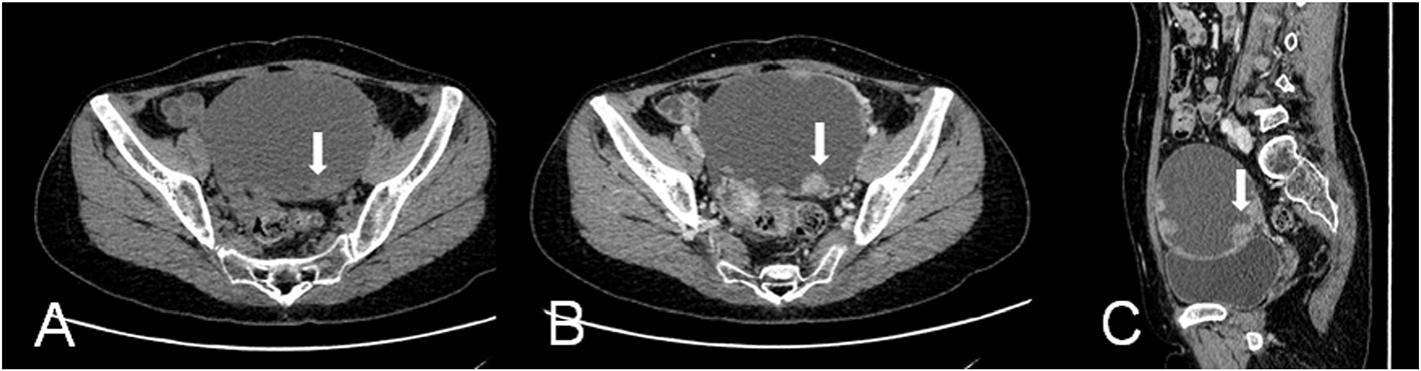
Ovarian clear cell carcinoma in a 47-year-old woman. Axial precontrast MSCT image (A) shows a mostly cystic, unilocular, and oval-shaped mass with smooth margin and round solid protrusion (arrow). Axial (B) and sagittal (C) contrast-enhanced CT images show obviously heterogeneous enhancement of the solid component in the tumor (arrow). The solid protrusions are both round and few in number.
